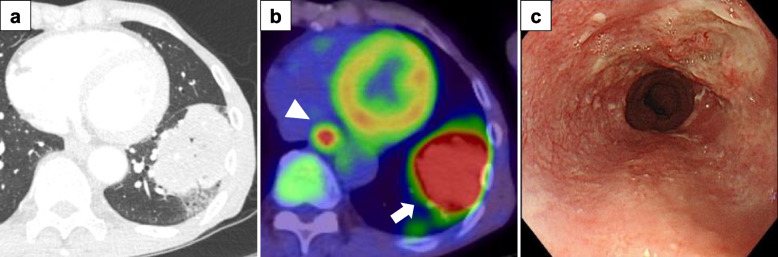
a CT image showing the solid tumor in the left lower lobe. b FDG-PET/CT image showing the accumulation of FDG in the lung tumor (arrow) and the lower thoracic esophagus (arrowhead). c Upper gastrointestinal endoscopy image showing a superficial, flat type of esophageal cancer
